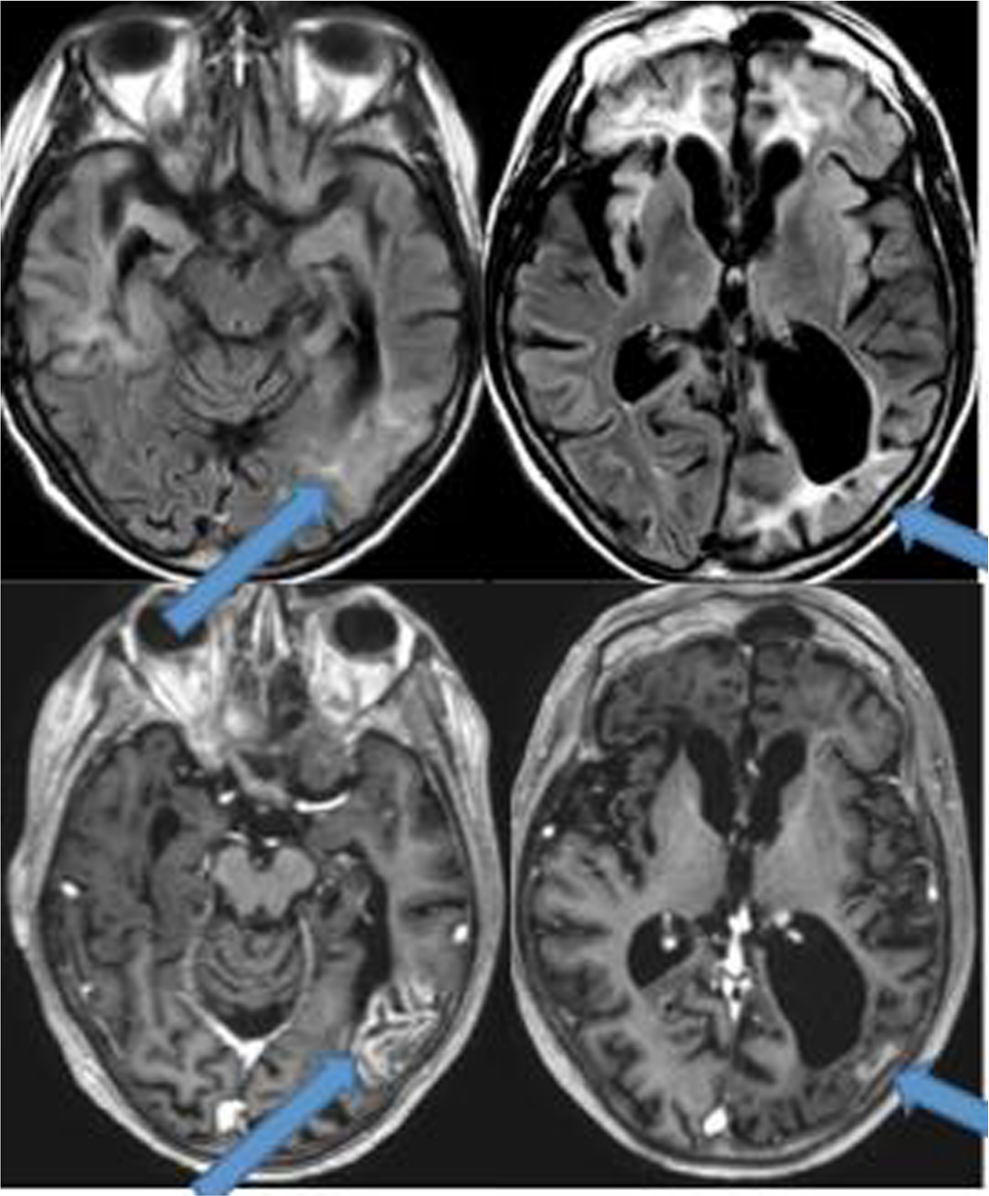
Case one, 2013: FLAIR hyperintensity has increased in the left parieto-occipital region (see *arrows*, *top images*), with new gyriform enhancement (see *arrows*, *bottom images*) on corresponding pre-contrast FLAIR sequences. The right basifrontal contrast enhancement has shown a complete resolution
